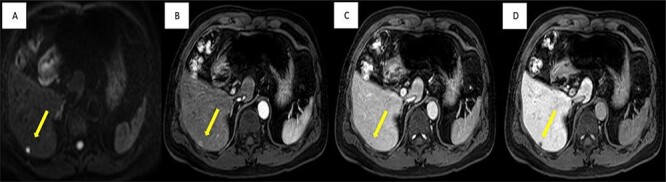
Imaging findings; MRI shows well-defined 6-mm nodule (yellow arrow), (A) strongly high signal intensity in diffusion-weight image, (C) slightly enhanced in arterial phase, (C) low signal intensity in portal phase and (D) non-uptake in hepatobiliary phase.
